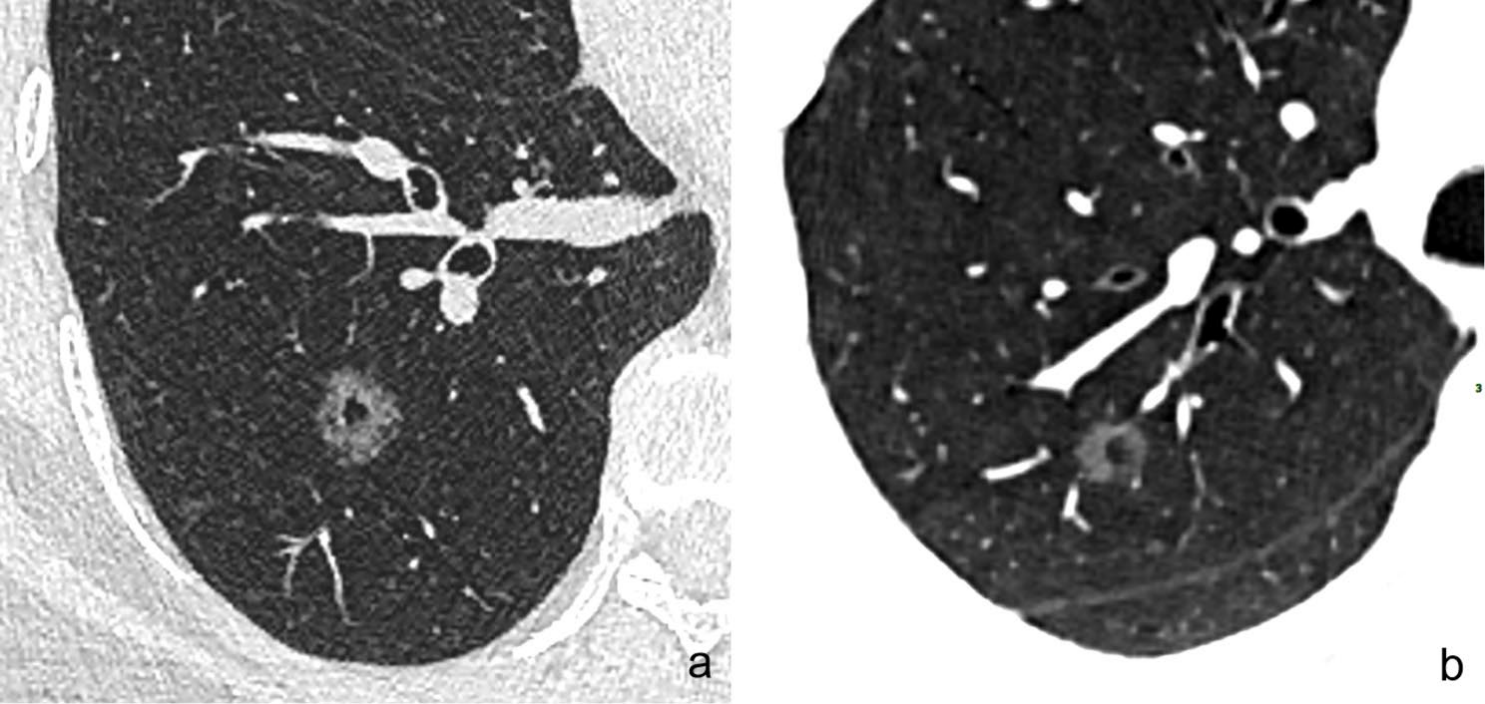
Different CT manifestations of the BLL sign in GGNs. (a) The density of BLL in the GGN (MIA) is lower than that of peripheral normal lung tissue and is similar to that of air attenuation. (b) The density of BLL in the GGN (AIS) is lower than that of peripheral tumor tissue, and which is similar to that of adjacent normal lung tissue. BLL: bubble-like lucency; GGNs: ground glass nodules; MIA: minimally invasive adenocarcinoma; AIS: adenocarcinoma in situ
